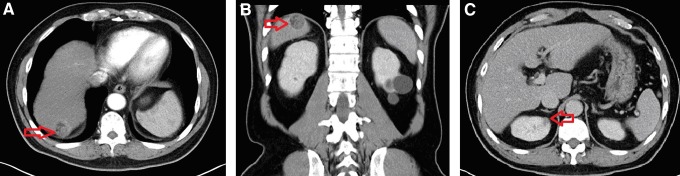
(A) Liver CT axial view: A 2 cm hypervascular tumor (*arrow*) was located at the S7 liver dome. (B) Liver CT coronary view: The tumor was located at the dorsal aspect of the liver dome. (C) A 1 cm renal tumor was located at the upper pole of the right kidney; we suspected that the tumor was renal-cell carcinoma.
